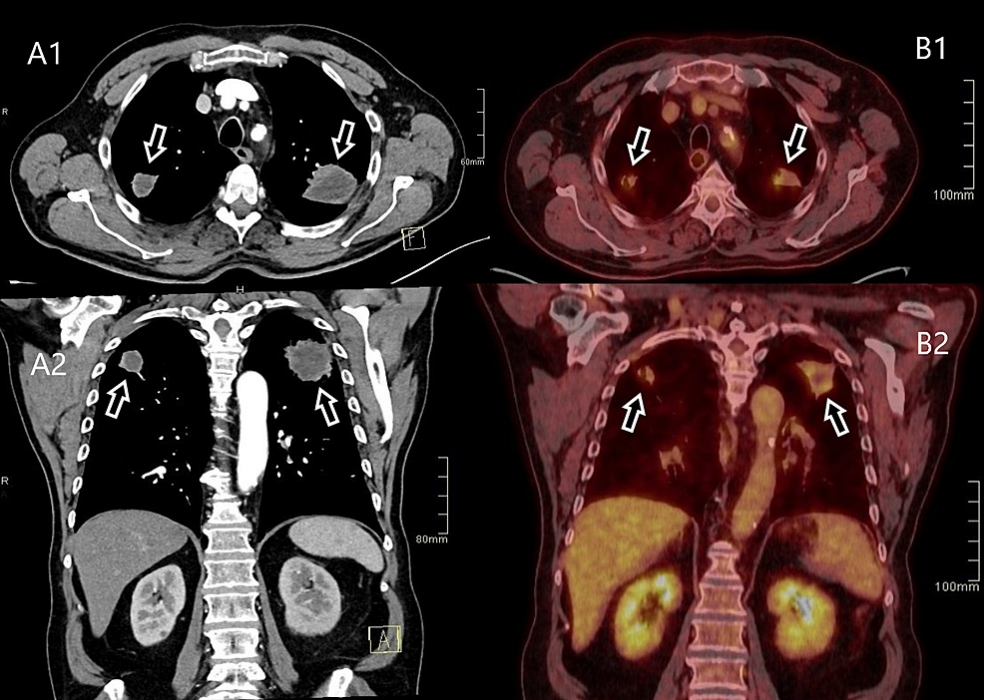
CT of the chest in the axial and coronal planes when the patient was first admitted to the hospital (A1, A2), and a follow-up PET-CT in the axial and coronal planes after two months of treatment, showing a decrease in lesion size (B1, B2). Lesions are marked with arrows. CT: computed tomography; PET-CT: positron emission tomography-computed tomography
